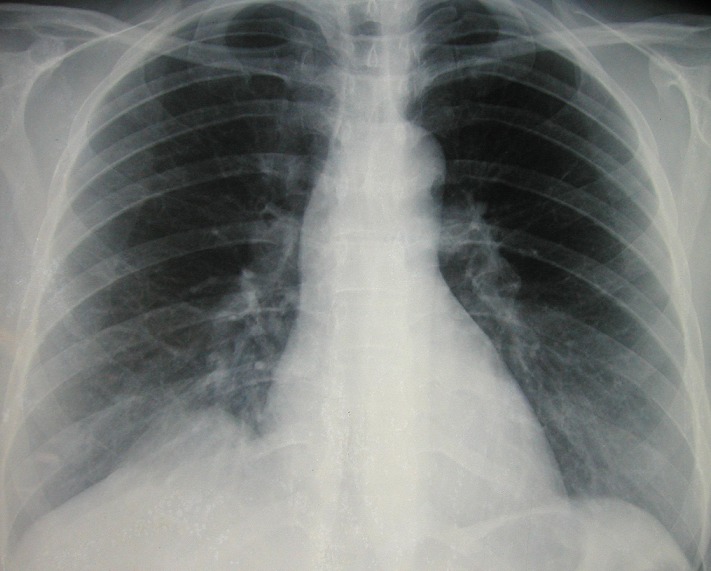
Radiographie thoracique de face montrant une opacité basale paracardiaque droite se confondant avec le diaphragme
